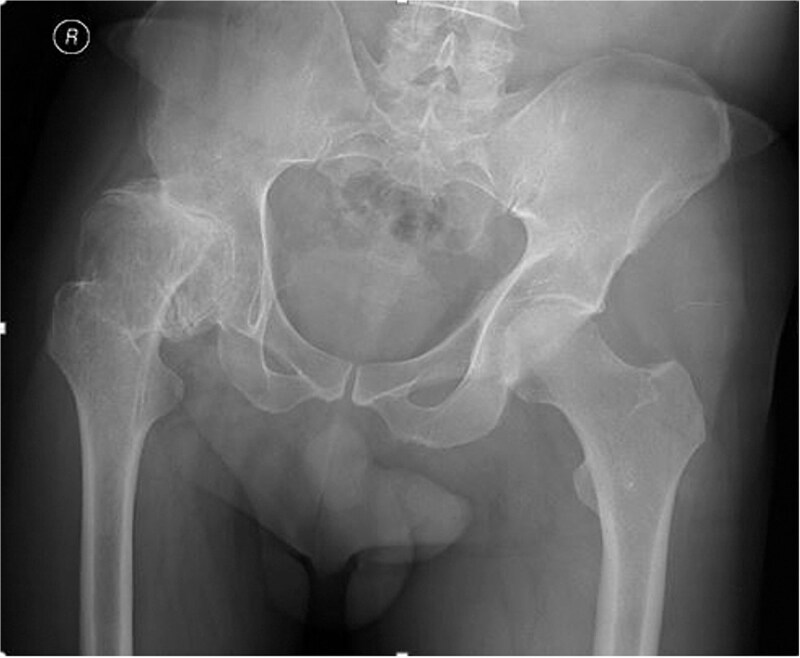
Preoperative anteroposterior radiograph of the pelvis demonstrating advanced right hip osteoarthritis (Tönnis 3), acetabular dysplasia (Crowe I), valgus hip, and pelvic obliquity.
